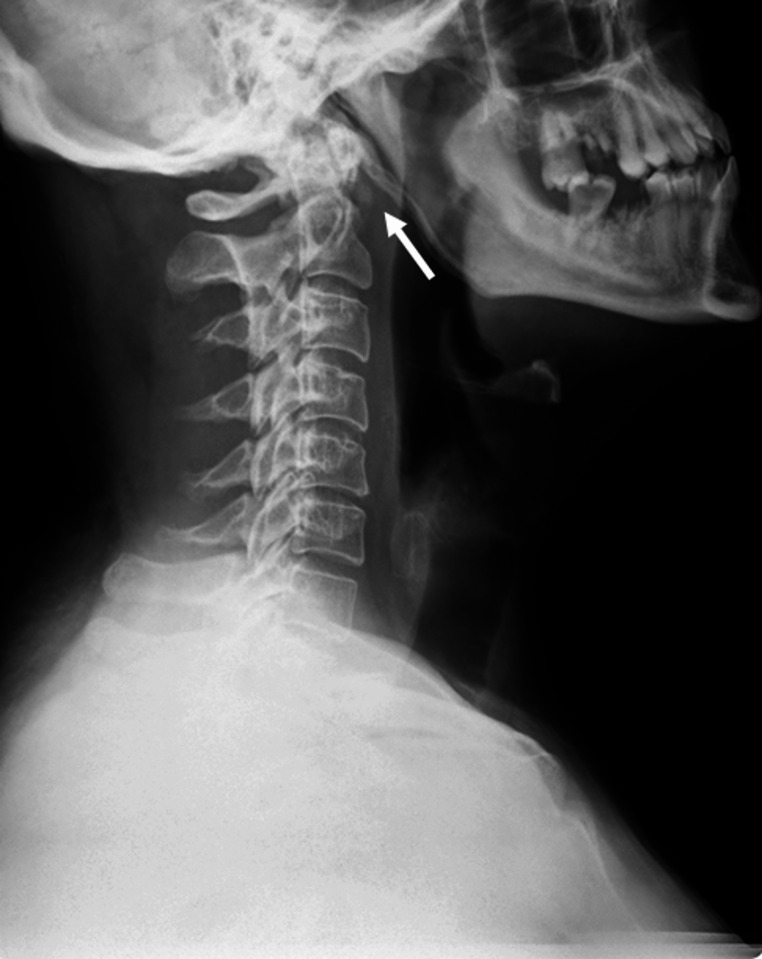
Lateral cervical spine radiography of a patient with *Longus Colli Calcific Tendinitis* where a calcific deposit (white arrow) is clearly identified on the anteroinferior border of the anterior arch of C1
